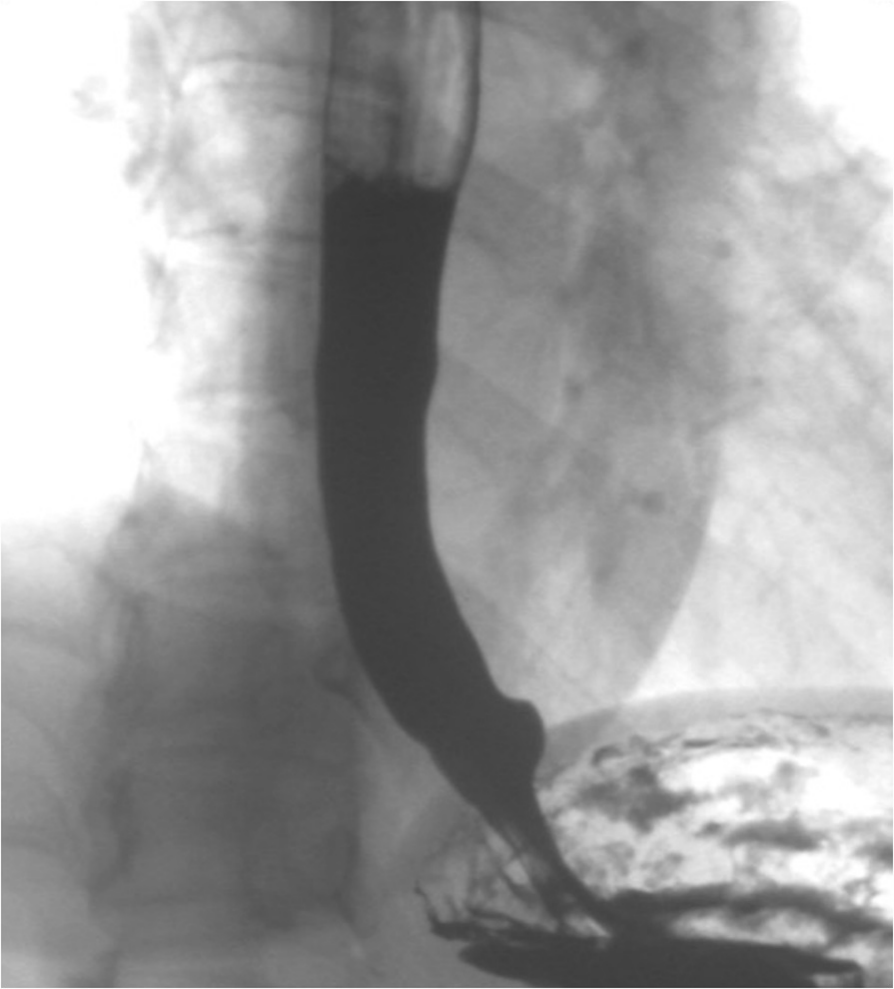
Radiograph of the barium esophageal transit examination. The findings have characteristic appearance of achalasia
